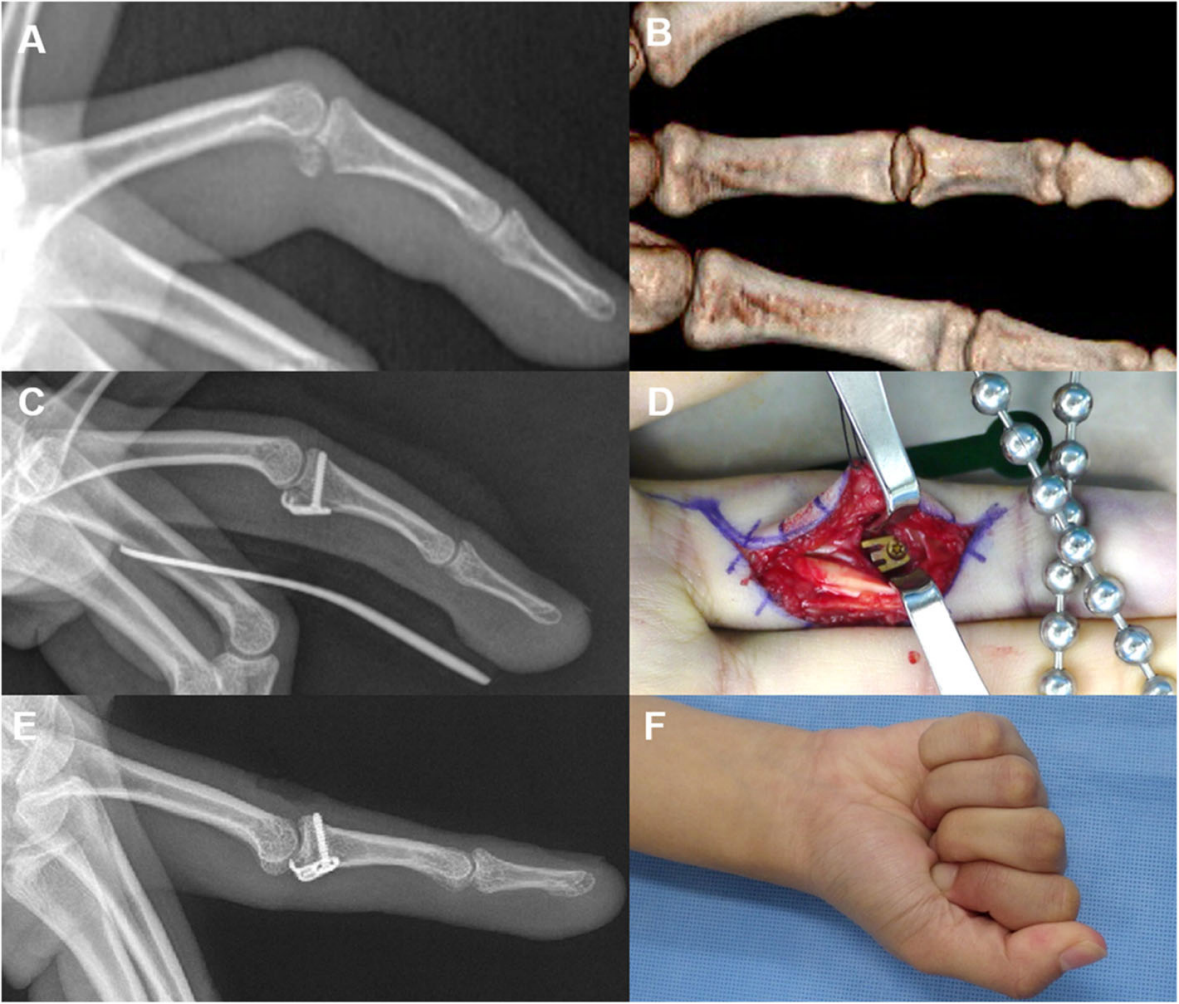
Volar plate avulsion fracture (a, b). The fragment was reduced, and a hook plate was placed over the fracture fragment, with the proximal hook end capturing the soft volar plate after opening the A3, C2, and C3 pulleys and then retracting the deep and superficial flexors (c, d). Plain radiography 55 months post-operation revealing that the osseous fragment was well united. The total range of MP joint motion was 100%, and the pinching strength was 90% compared to the values of the same contralateral joint (e, f)
